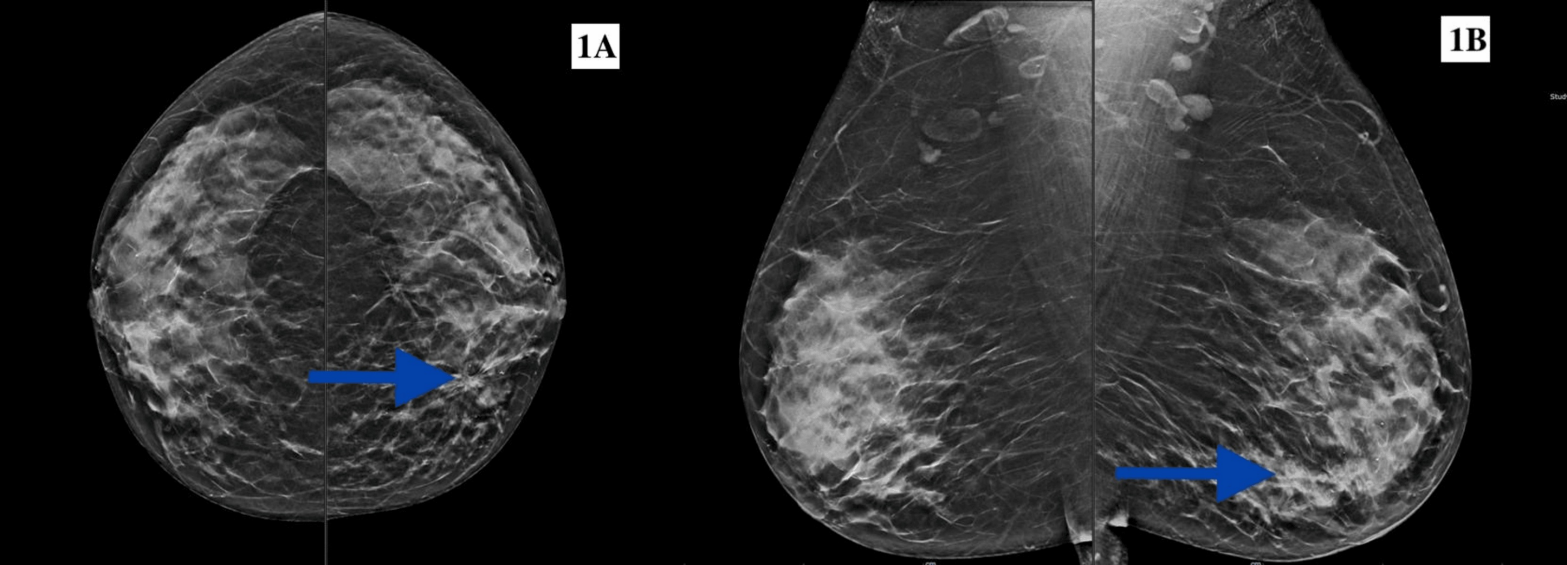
Mammography showing mediolateral (A) and craniocaudal view (B). The blue arrow shows an ill-defined mass with indistinct margins in the central quadrant of the left breast with associated architectural distortion. Multicentricity was better appreciated on ultrasound breast images.
